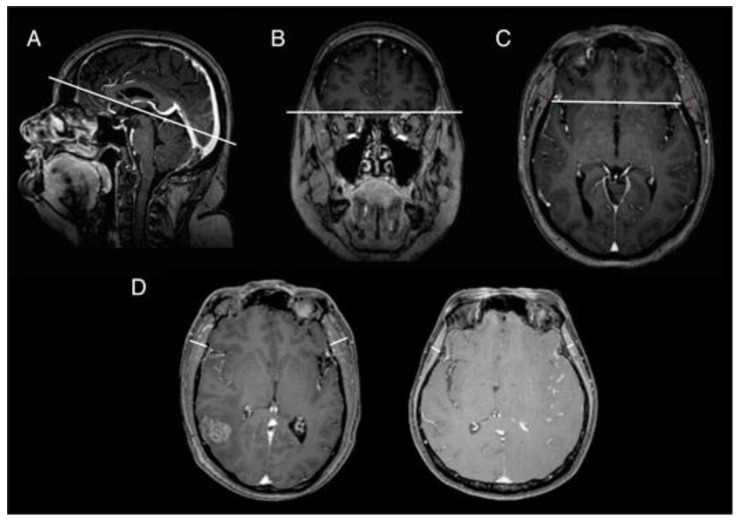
Example of TMT measurements using standard isotropic contrast-enhanced T1-weighted cranial MRI (A–C) demonstrate the MRI alignment for TMT acquisition. (D) A 57-year-old male without sarcopenia (left), a 48-year-old female with sarcopenia (right). MRI, magnetic resonance imaging; TMT, temporal muscle thickness.
